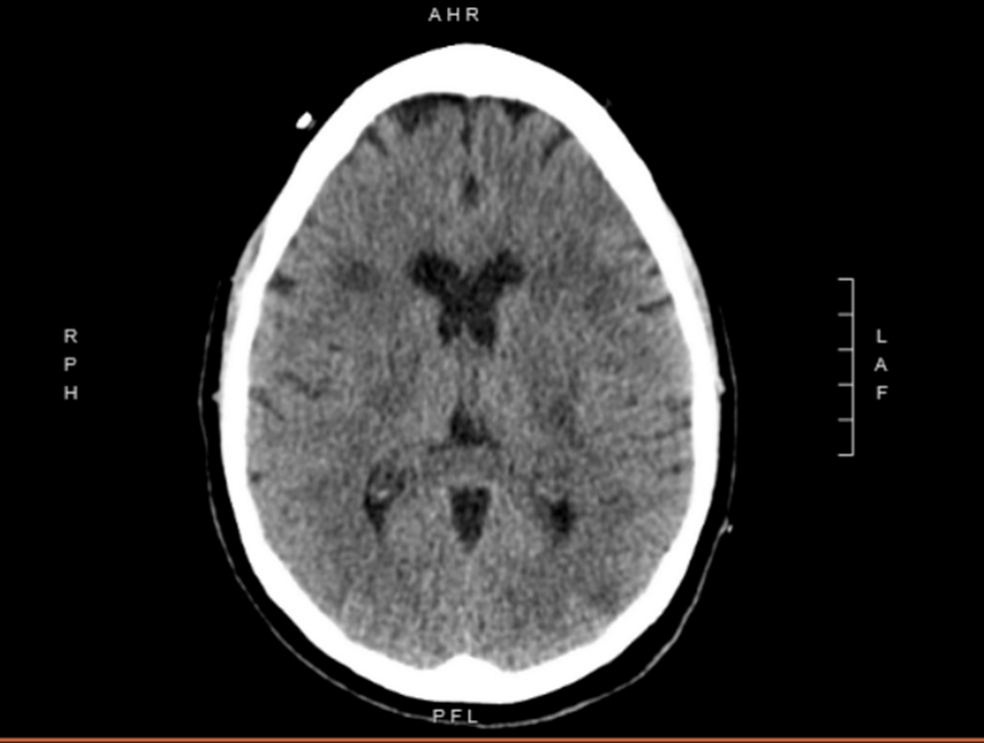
CT scan showing confluent multifocal white matter and basal ganglia hypoattenuation lesions. CT, computed tomography
